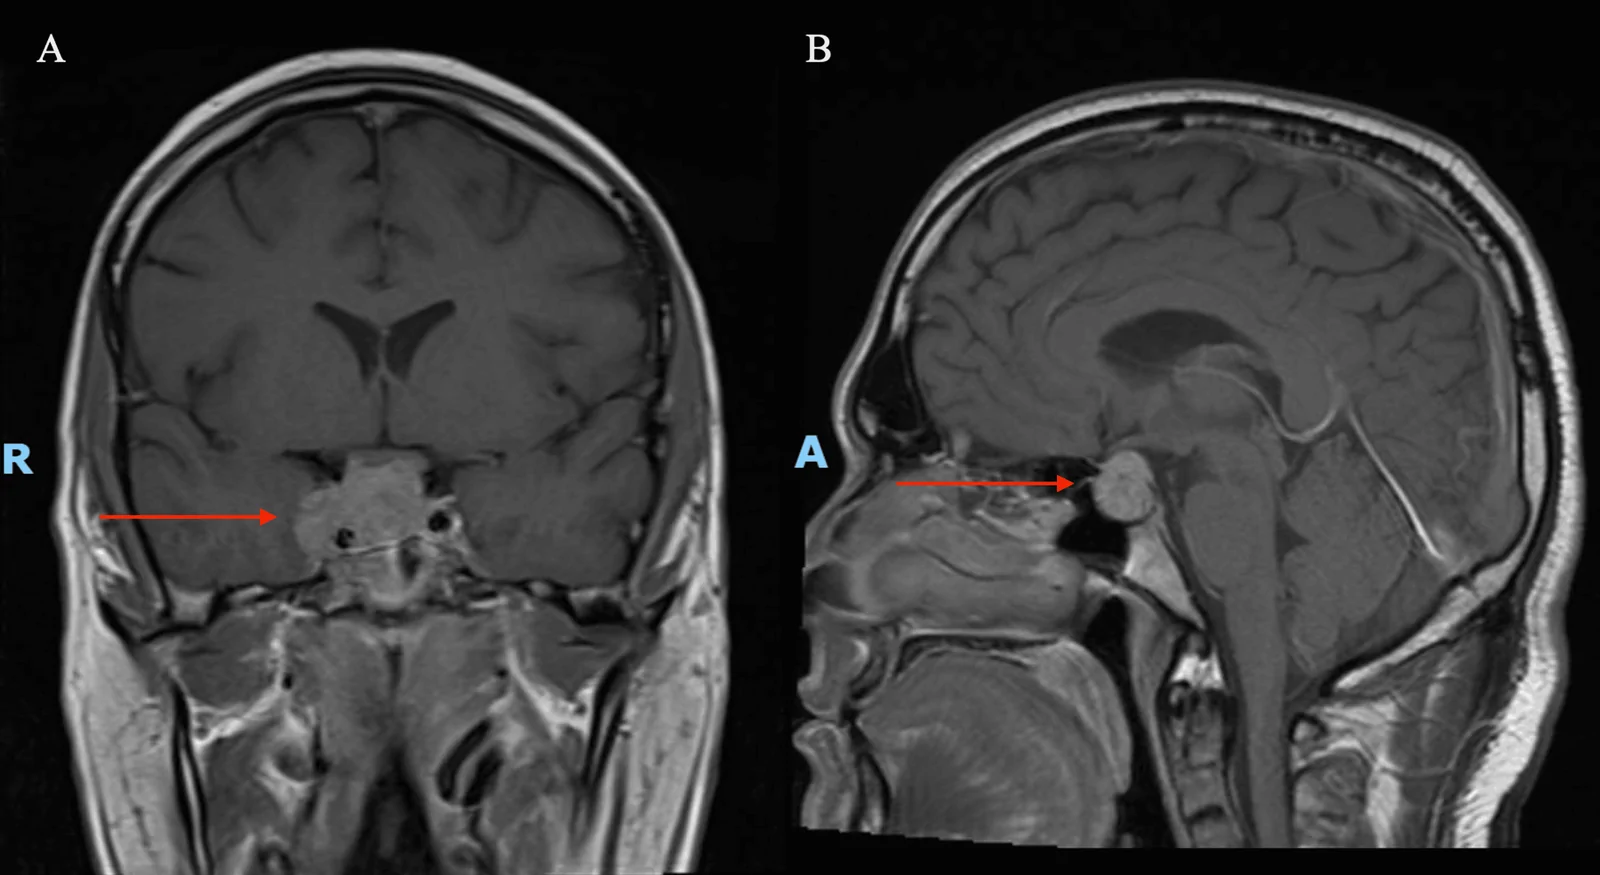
Preoperative images (T1-weighted with contrast coronal (A), sagittal (B)) showing a mildly enhancing pituitary macroadenoma approximately 2.8x2.0x1.8 cm extending into the right cavernous sinus encasing the right cavernous internal carotid artery and slightly into the left cavernous sinus with abutment of the left cavernous internal carotid artery. There is also suprasellar extension with slight lifting of the optic chiasm.
